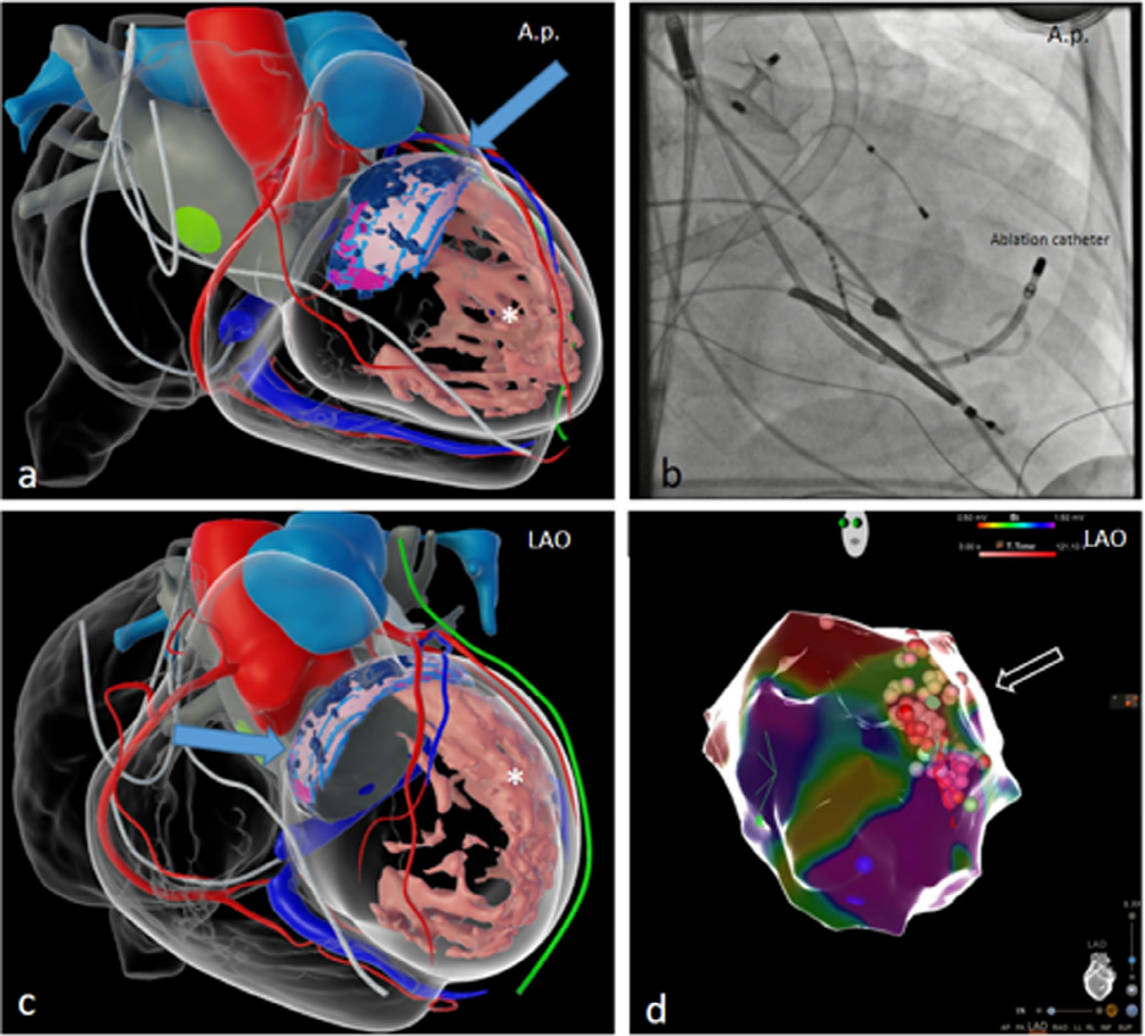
Inheart CT scans, fluoroscopy and intracardiac 3D map. Segmented Inheart CT, angulation a.p. (a) and LAO (c). Segmentation revealed the prior ablation site (blue arrow) and extensive intramural fibrotic tissue (asterisk). (b) fluoroscopic view of the ablation catheter position in a.p. (d) intracardiac 3D map with ablation sites, matching the intramural fibrotic tissue.
